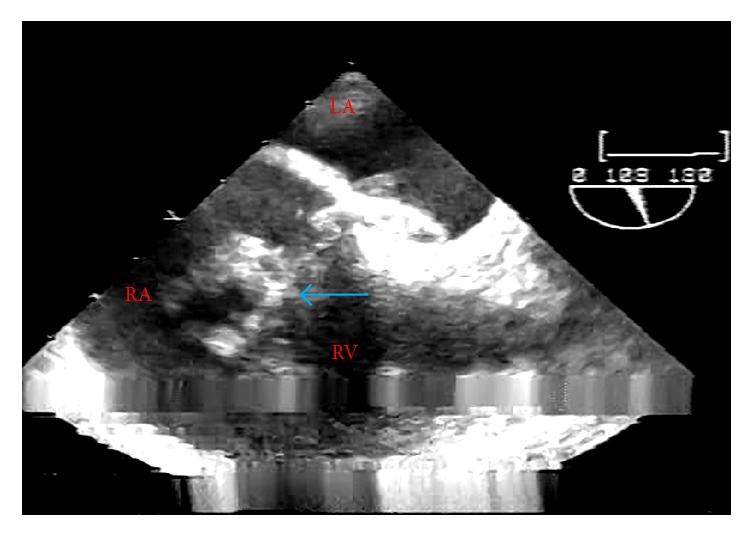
Initial intraoperative transesophageal echocardiography; midesophageal 4-chamber view. A large right atrial thrombus was visualized (blue arrow). LA, left atrium; RA, right atrium; RV, right ventricle.
